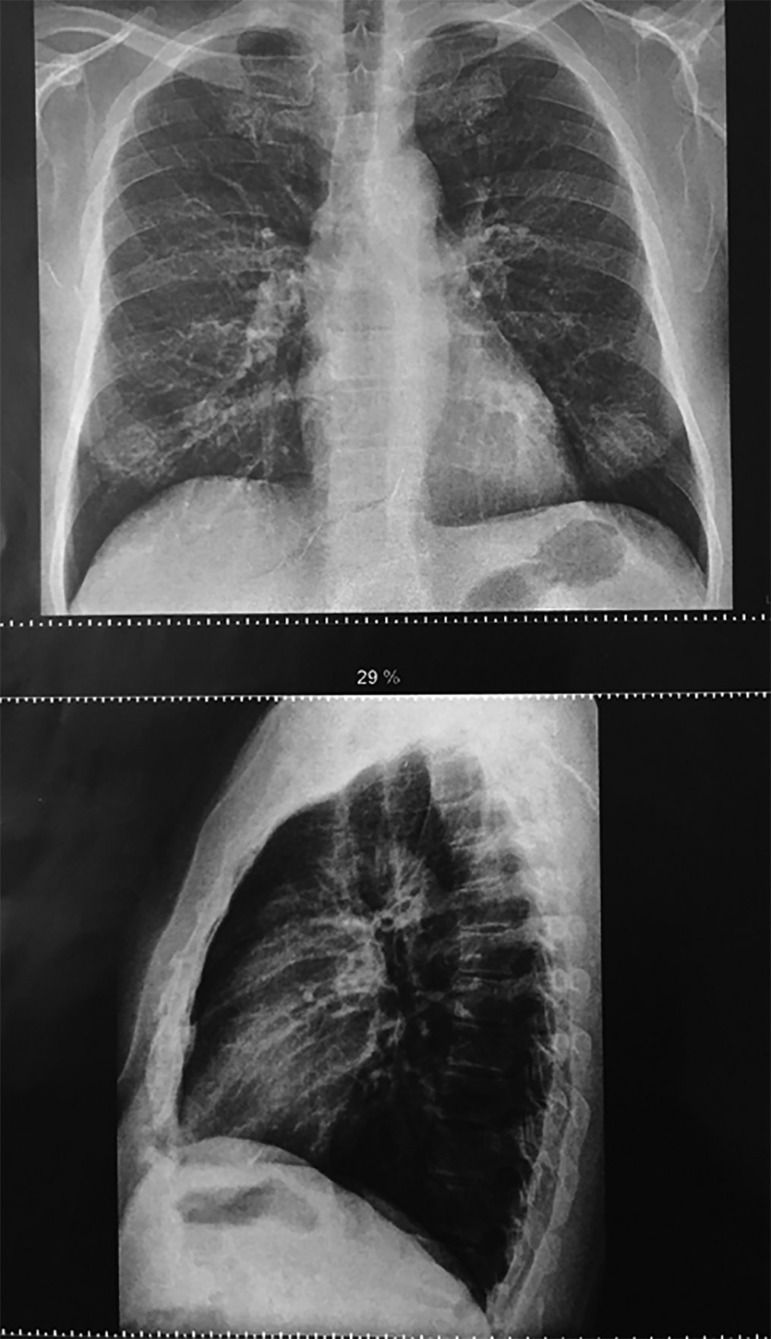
Chest X-ray: outpatient follow-up.
